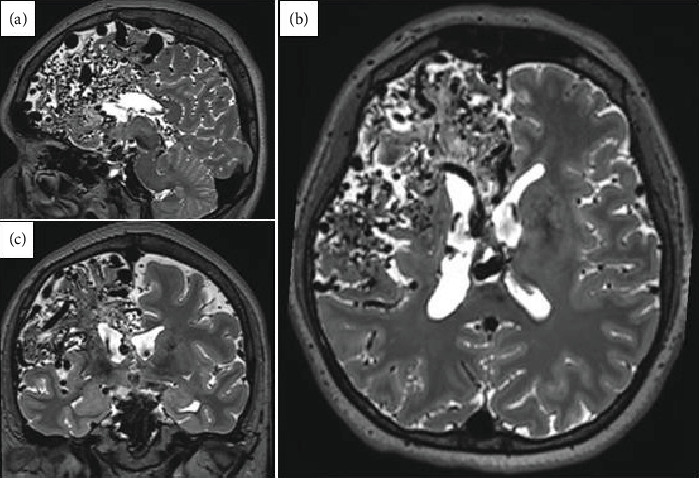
3.0 T brain MRI T2 sequence performed at Age 6 revealing a large 11 cm AVM in the right frontal lobe, extending to the insular and parietal regions. The AVM receives arterial supply from the right middle cerebral artery and the anterior cerebral arteries, compromising the perirolandic region. Additional findings include areas of gliosis and hemosiderin deposition. (a) Sagittal view. (b) Coronal view. (c) Axial view.
